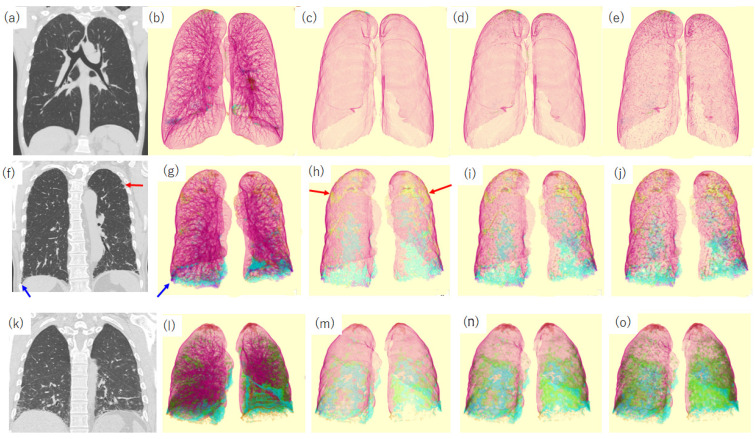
Coronal CT image and quantification CT images of the lung surface. (a–e) Images of a 41-year-old male never-smoker with normal respiratory function. (f–j) Images of a 70-year-old male patient with IPF (images from the same patient are shown in Figure 10, Figure 11 and Figure 12). (k–o) Images of a 41-year-old man with pathologically proven idiopathic NSIP. (a,f,k) Coronal CT images obtained at standard dose. (b,g,l) Segmented images of the entire lung. (c,h,m) outer part with a 2 mm width. (d,i,n) outer part with a 5 mm width. (e,j,o) outer part with a 10 mm width. All segmentations were performed using QZIP-ILD. Segmentation color codes are shown in Figure 10. In healthy individuals, no structures are observed in the outer part, with a width of 2 mm. (c) Peripheral pulmonary vessels and bronchi are recognized in the outer part with a 10 mm width. (e) In patients with UIP, a violet pattern, indicating a honeycomb is observed in the bilateral lung base (blue arrows in f and g). Additionally, a yellow pattern corresponding to subpleural fibrosis is observed on the surface of the bilateral upper lobes (red arrows in f and h). In patients with NSIP, the upper lobe surface area shows a normal pattern. Abbreviations: CT, computed tomography; IPF, idiopathic pulmonary fibrosis; NSIP, nonspecific interstitial pneumonia; and UIP, usual interstitial pneumonia.
